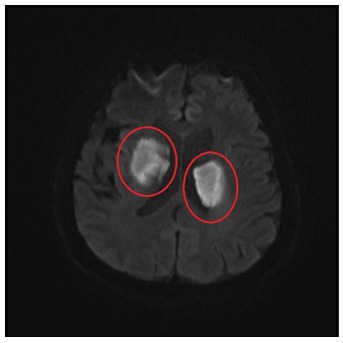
Hyperintense areas in bilateral suprathalamic regions in B1000 sequence on diffusion-weighted MRI.
